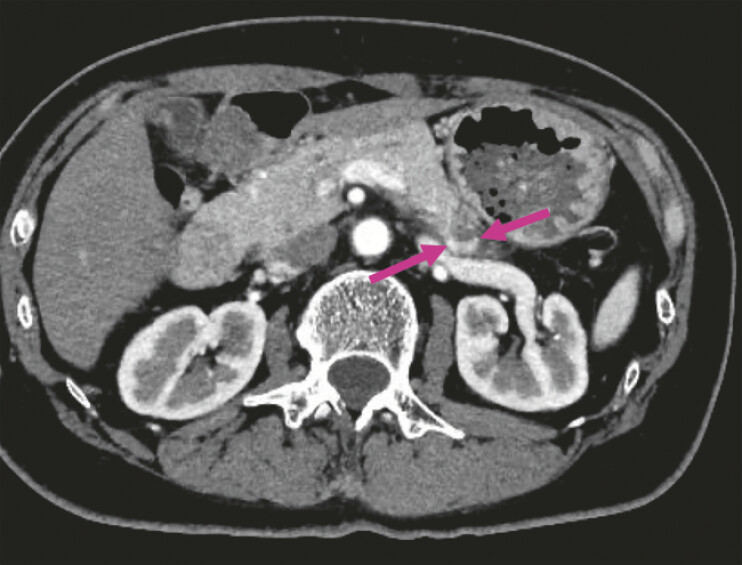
An early-enhancing area (arrow) at the lesion margin on contrast-enhanced computed tomography performed 3 days after the procedure.
